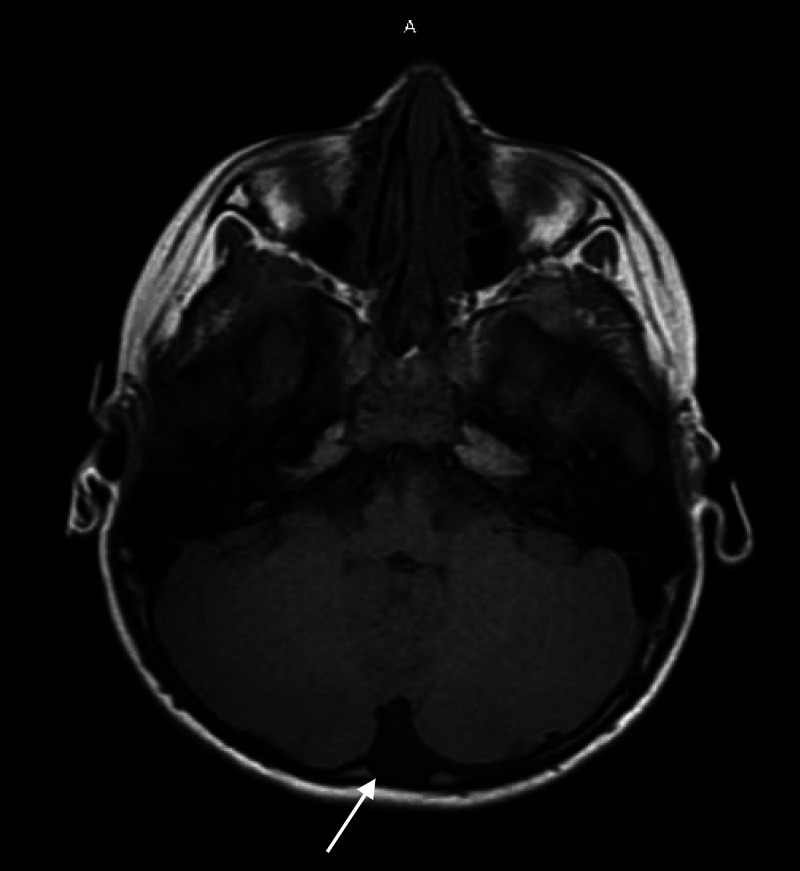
MRI showed a small arachnoid cyst in the left posterior fossa of the left cerebellar hemisphere
